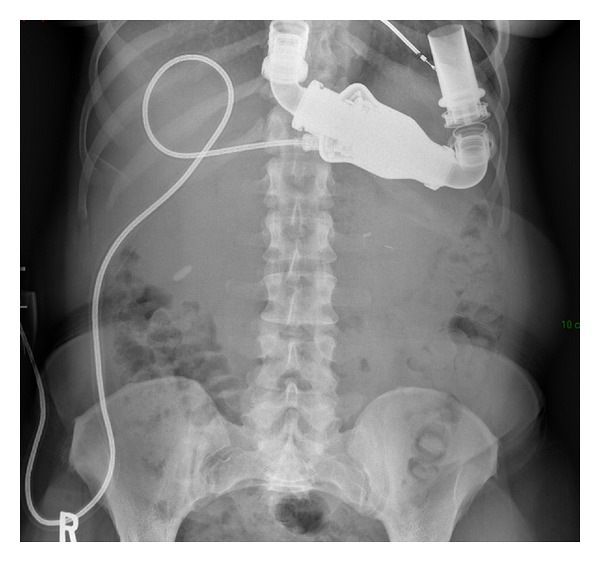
Confirmation of no retained sponge with series of abdominal plain films.
